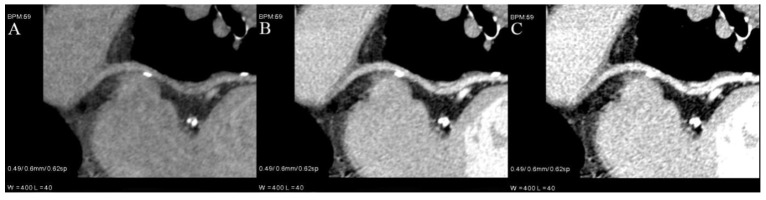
Curved VMI reconstruction of coronary graft of poorly enhanced CTA. Three reconstructions with different energies ((A)—80 kev, (B)—60 keV, (C)—50 keV) and the same window and level settings. Contrast density is rising with lower energies, but also noise and artifacts from metal clips around graft.
